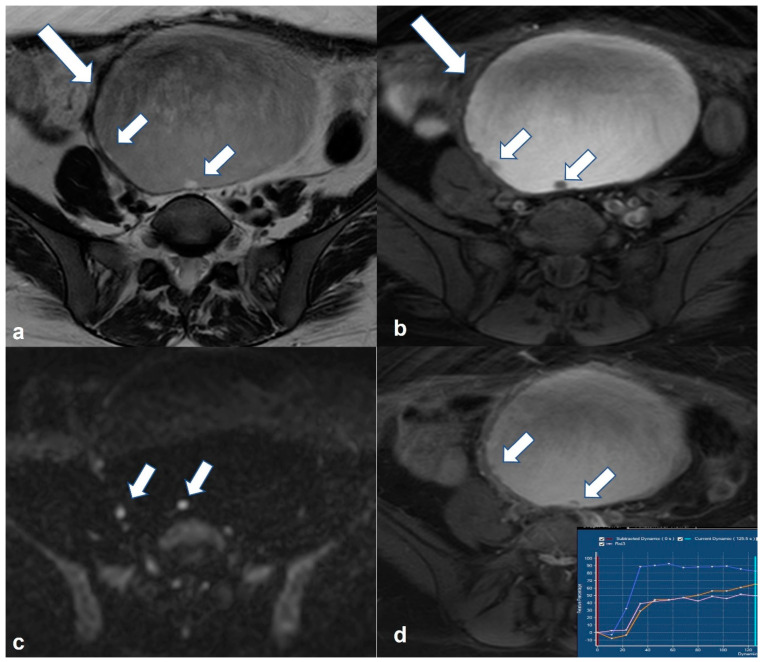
Endometrioid borderline ovarian tumor Axial T2 weighted image (a) shows a cystic mass (large arrow) originating from the right ovary with hemorrhagic content on corresponding axial T1 weighted FS image (b). There are small mural nodules in the right posterolateral aspect of the lesion (small arrows in a and (b)) with restricted diffusivity on corresponding axial high b value DWI image (small arrows in (c)). Note the enhancement of the nodules on the corresponding axial T1 weighted FS CE image (small arrows in (d)). DCE analysis showed a type 2 time–intensity curve (inset in (d), blue line: uterus; yellow/pink line: nodules).
